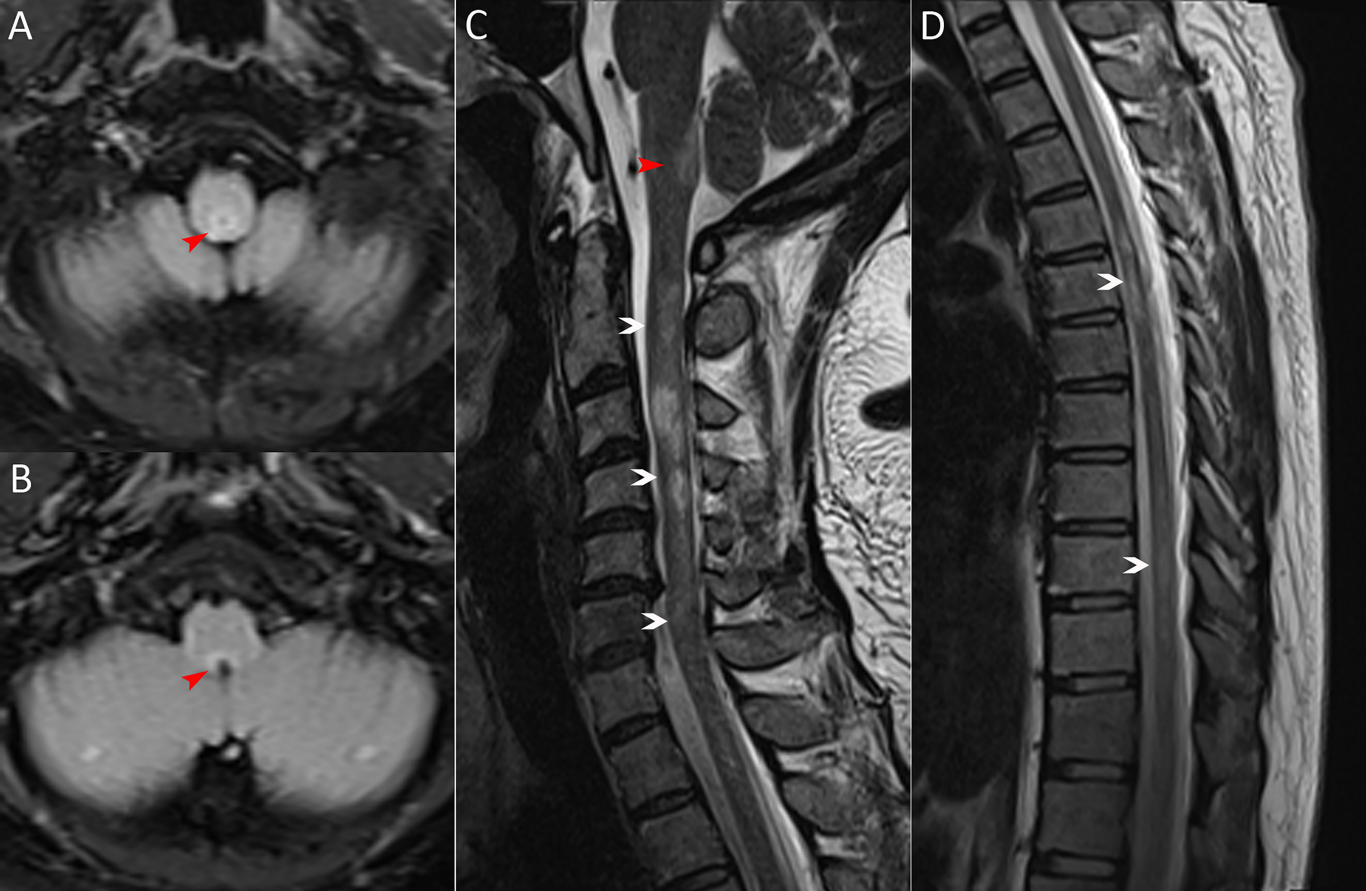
MRI scans on admission. (A, B) Brain MRI (axial fluid-attenuated inversion recovery image) exhibiting the area postrema lesion (red arrows, including one in Figure C). (C, D) Spinal cord MRI (sagittal T2-weighted image) showing demyelinating lesions in the cervical and thoracic segments (white arrows).
